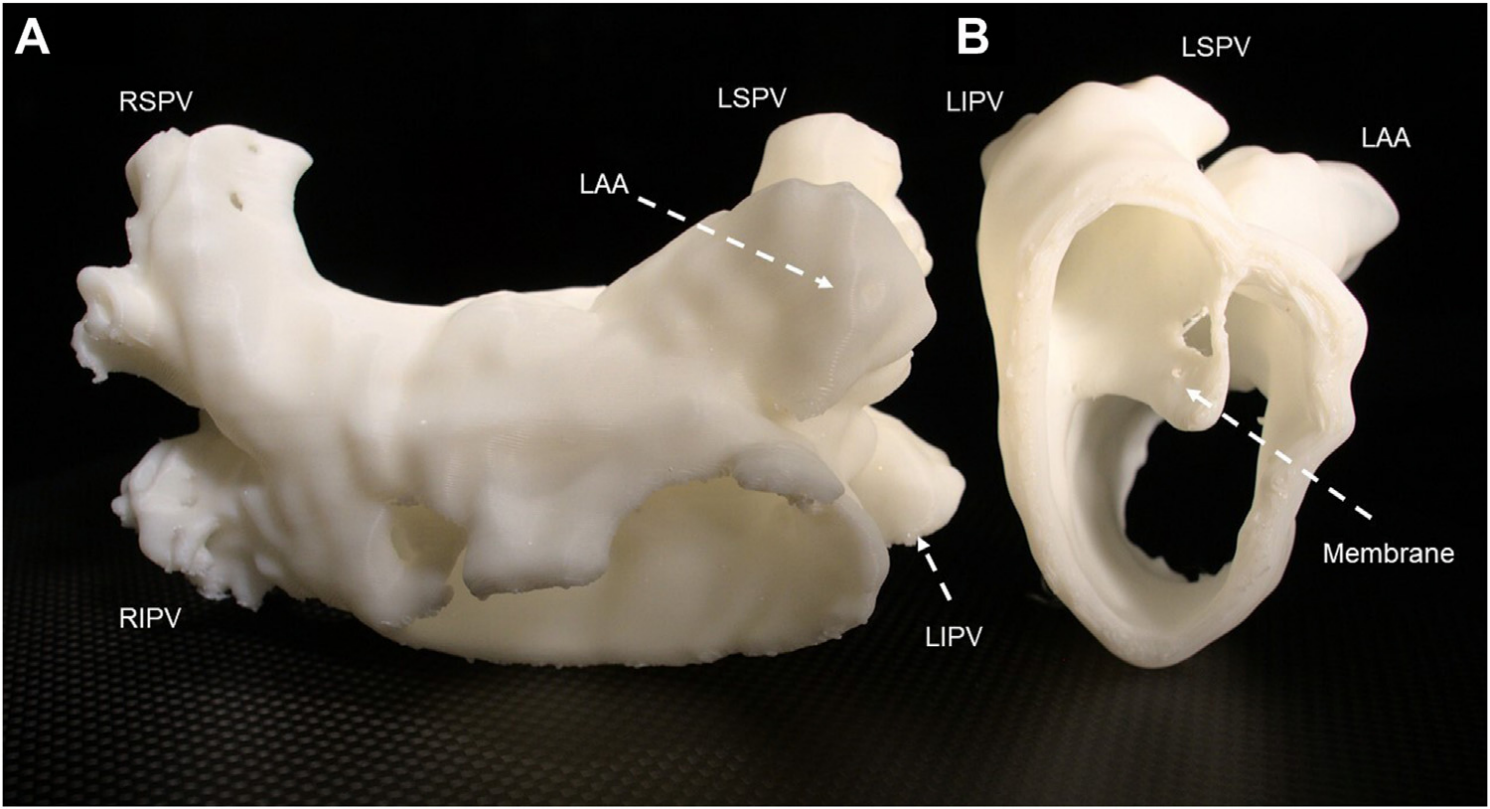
A model of the left atrium using the multidetector computed tomography data created via a 3D printer. A: Anterior-posterior view. B: Sagittal view. LAA = left atrial appendage; LIPV = left inferior pulmonary vein; LSPV = left superior pulmonary vein; RIPV = right inferior pulmonary vein; RSPV = right superior pulmonary vein.
